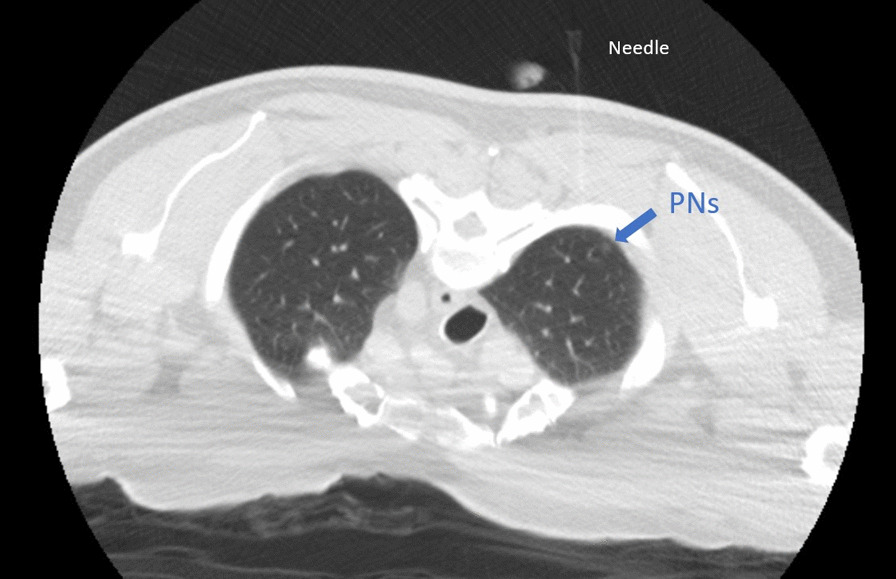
CT film of preoperative location
